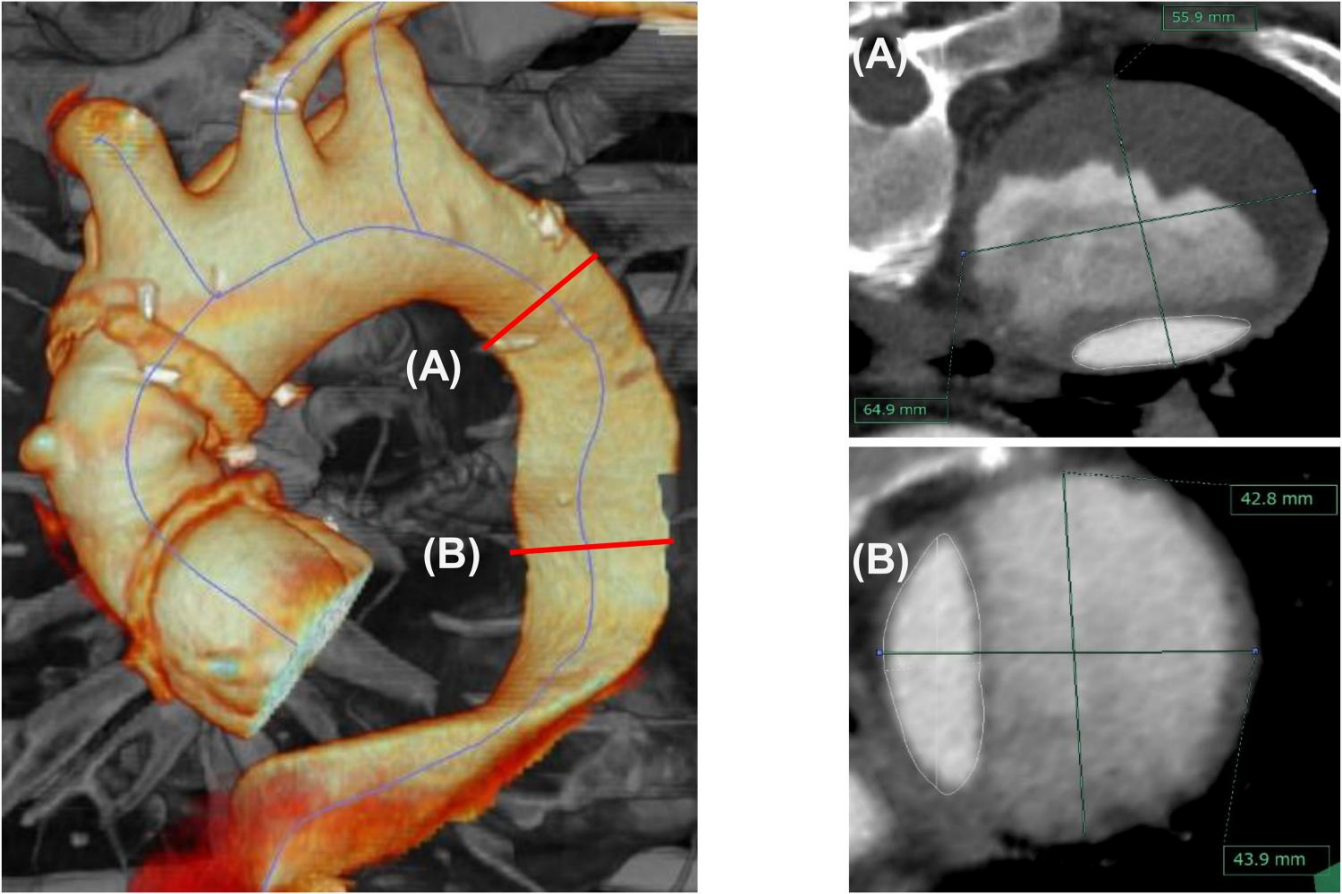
Standardized Aortic Measurement Levels. Left: 3D CT angiography showing level A in the proximal descending thoracic aorta (Ishimaru zone 3, 20 mm distal to the left subclavian artery) and level B in the mid-descending thoracic aorta (Ishimaru zone 4, at the level of the left lower pulmonary vein). Right: corresponding centreline-orthogonal cross-sections at level A (top) and level B (bottom) with outer-to-outer maximal aortic diameters.
